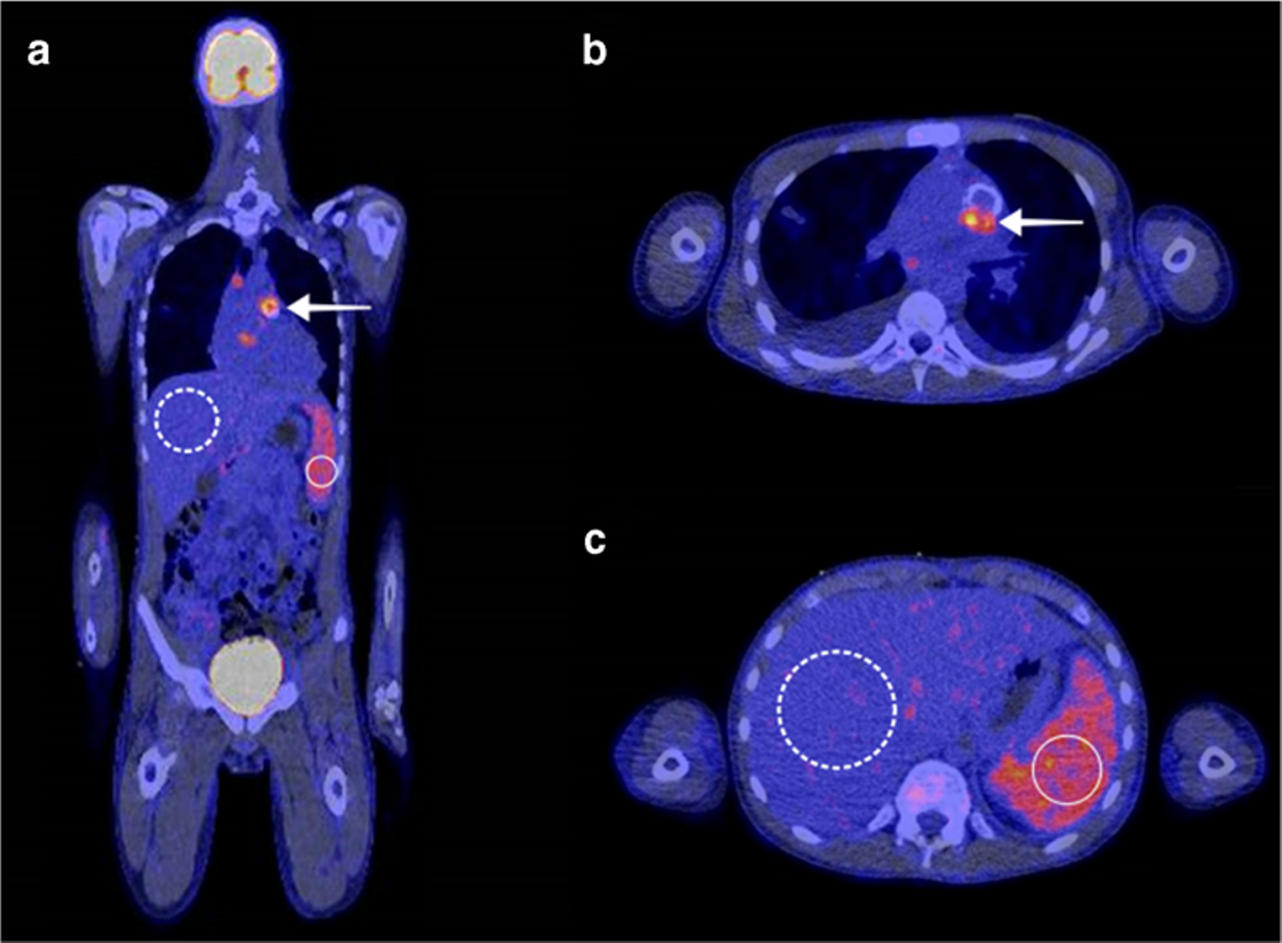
A 14-year-old boy presented to the hospital with an intermittent fever up to 40 °C, fatigue, and painful muscles in his legs for approximately 2 weeks. His medical history included aortic valve replacement with an artificial valve. On auscultation, a systolic murmur was heard, which was loudest at the third intercostal space on the left side of the sternum. His leukocyte count was 13.2 × 10^9^/L, and his CRP level was 40 mg/L. Blood cultures were positive for *Streptococcus parasanguinis*. A possible aortic valve vegetation was seen on a transthoracic ultrasound. On FDG-PET/CT, pathologic FDG uptake of the aortic valve was seen (A, B, white arrow), confirming the diagnosis of artificial valve endocarditis. FDG uptake in the spleen (white circle) was evidently higher than in the liver (dashed white circle), with an SLR of 1.73 and a BLR of 1.11. Despite adequate antibiotic treatment, there was no clinical improvement, and the aortic valve was surgically replaced 2 weeks after FDG-PET/CT. Six weeks after the surgery, the patient could be discharged from the hospital in good clinical condition
